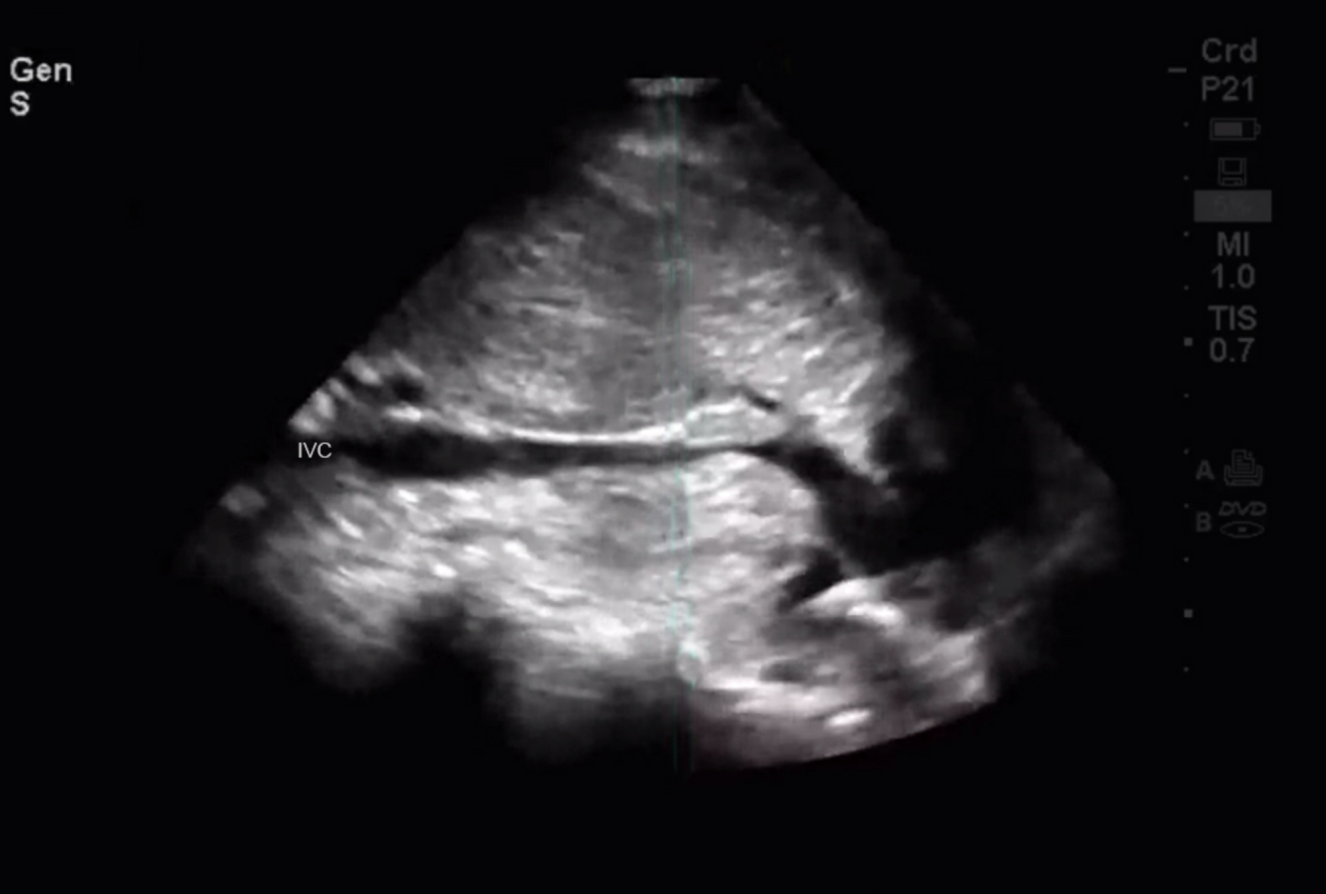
Ultrasonographic image of patient's patent inferior vena cava.
